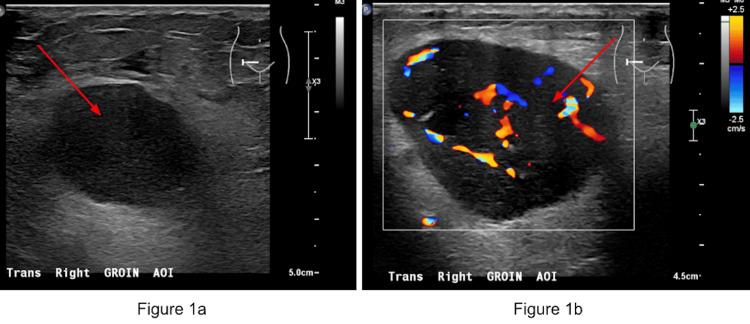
Pelvis limited non-obstetrical ultrasound of the right groin (red arrows) without Doppler (a) and with Doppler (b). The image demonstrates the right inguinal region with a vascularized, hypoechoic mass measuring up to 5.4 cm (red arrows).
